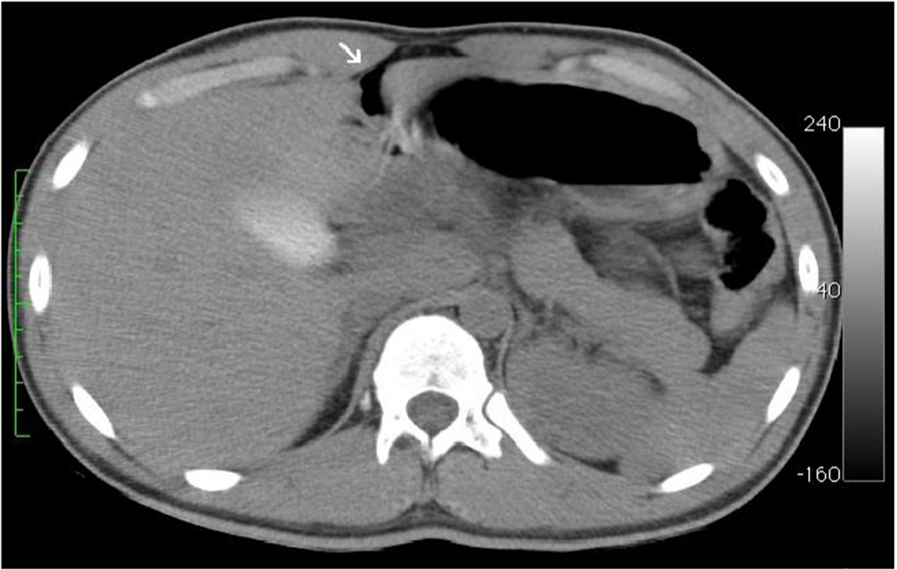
Repeated CT scan. A CT scan was repeated after the patient developed peritonitis. Peritoneal free air was detected (arrow).
